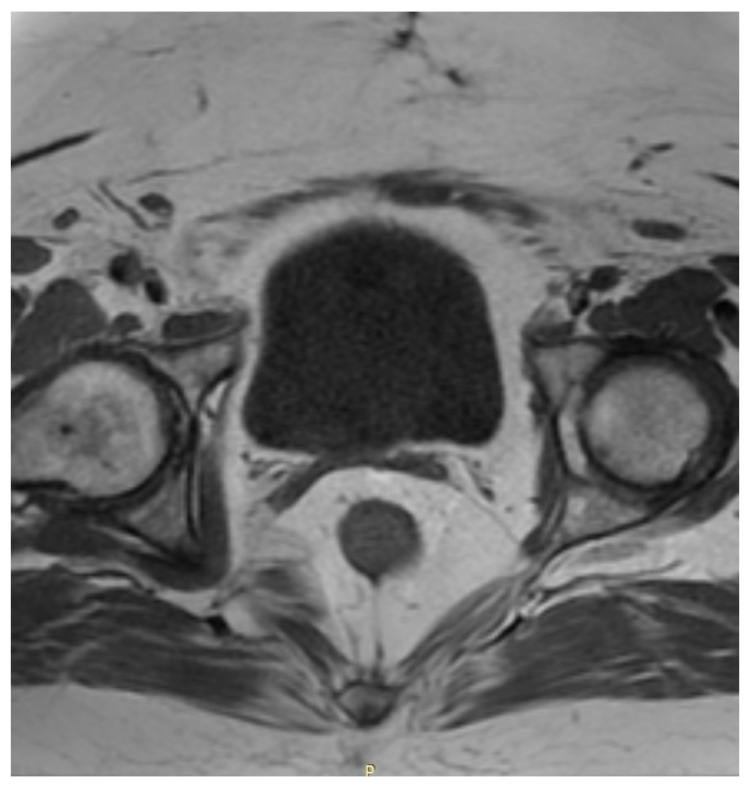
A postoperative MRI of the pelvis in axial projection, performed 18 months after the Wertheim–Meigs operation. A vaginal stump is visible. No signs of recurrence are present.
